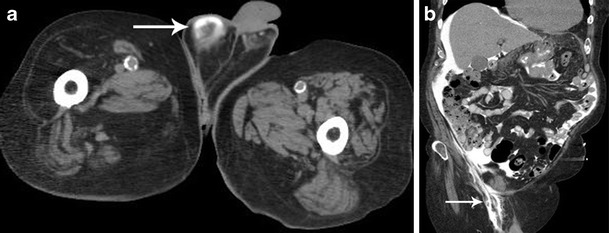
CT peritoneography axial (a) and oblique coronal (b) images demonstrate dialysate leakage via a patent right processus vaginalis into the right scrotal sac (*arrows*)
